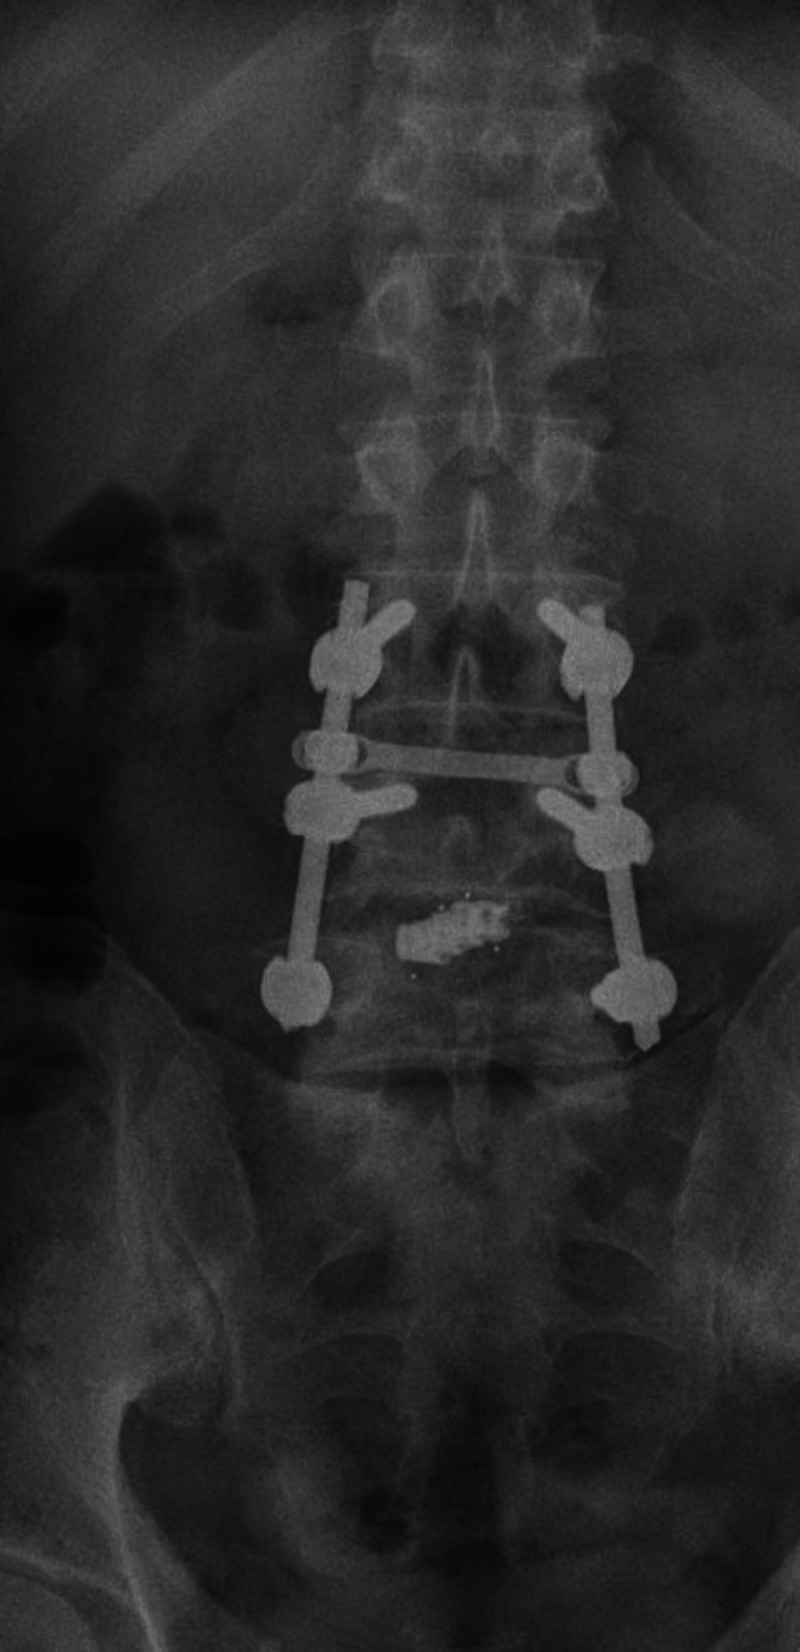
Postoperative anteroposterior standing lateral roentgenogram of the lumbar spine in the last evaluation, four years postoperatively.
